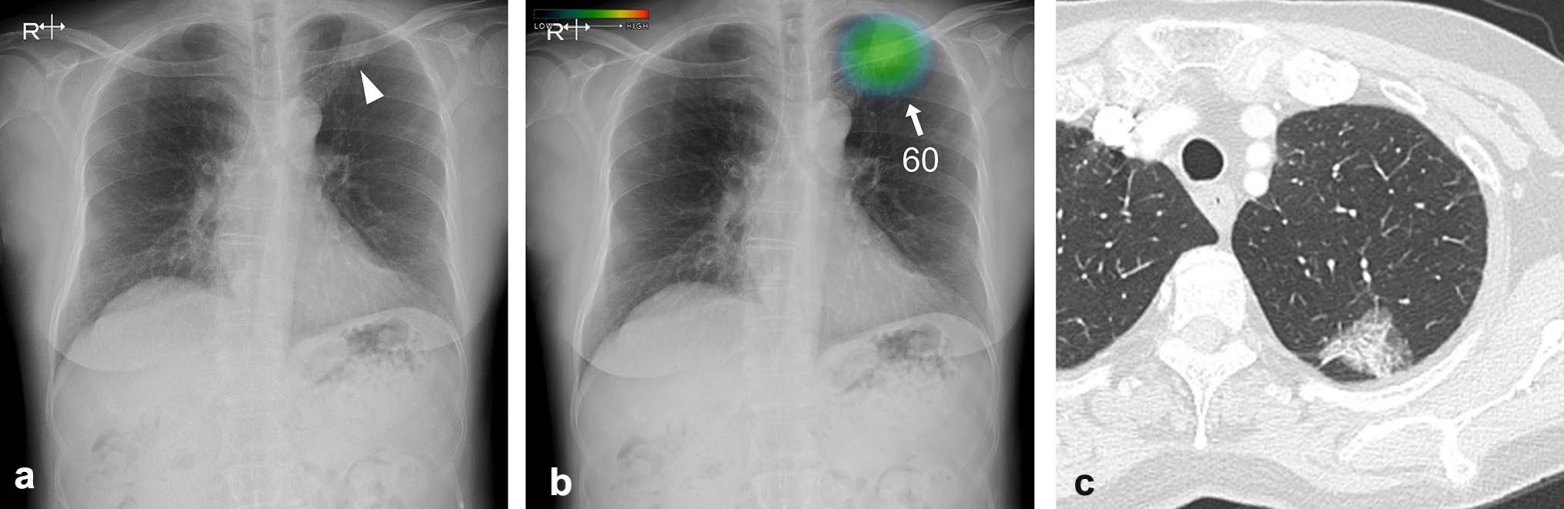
Diagnostic images of 74-year-old female patient with adenocarcinoma in the left upper lung. a Chest radiograph showing a nodule in the left upper lung masked by the left clavicle and first rib (arrowhead), with a visibility score of 3 and a background lung score of 2. b The nodule was properly detected by CAD with a probability of 60. c Chest computed tomography showing a part-solid nodule with a solid part of 2.2 cm in size
